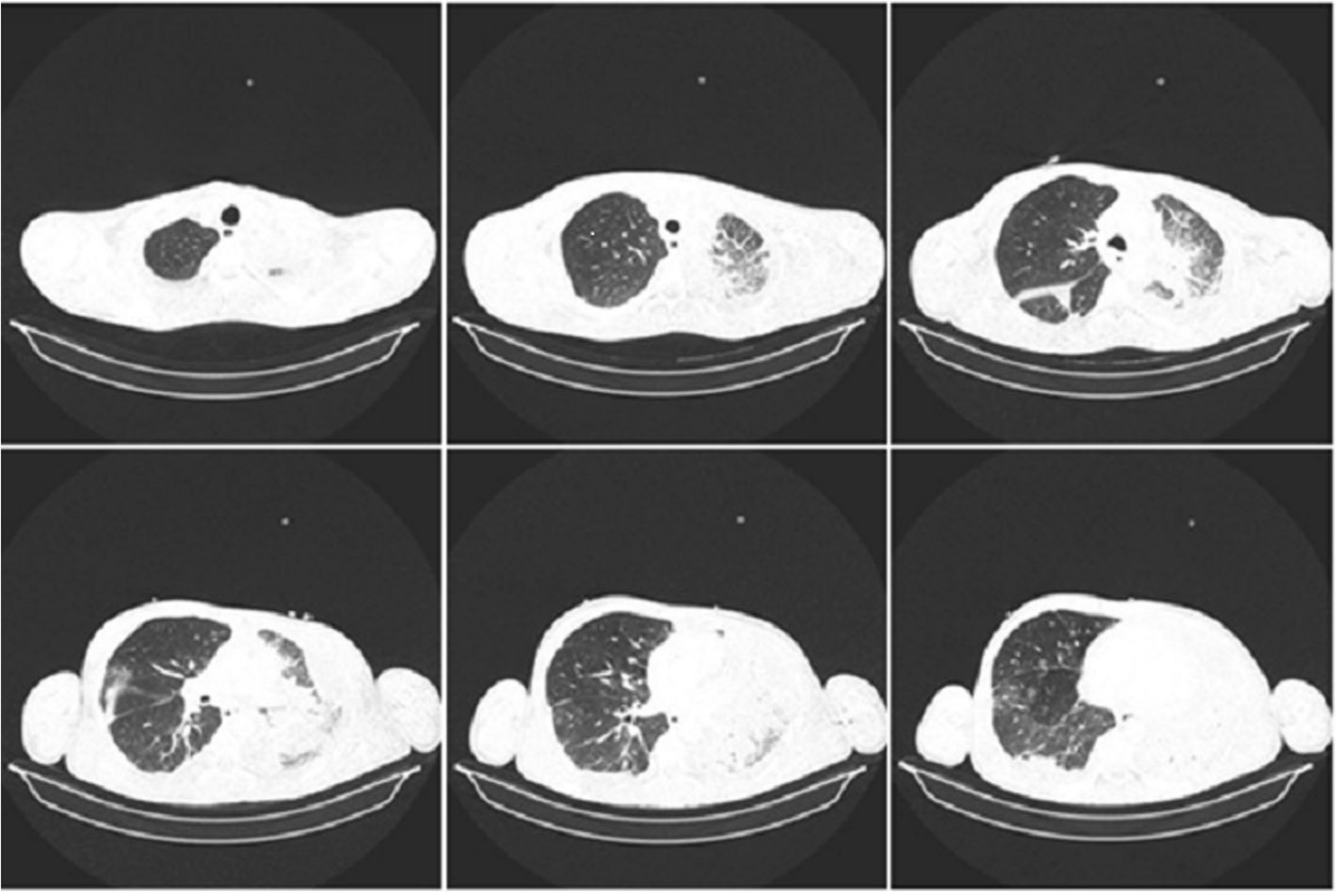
Computed tomographic scan of the chest of the patient on the 34th day of his stay in the intensive care unit
